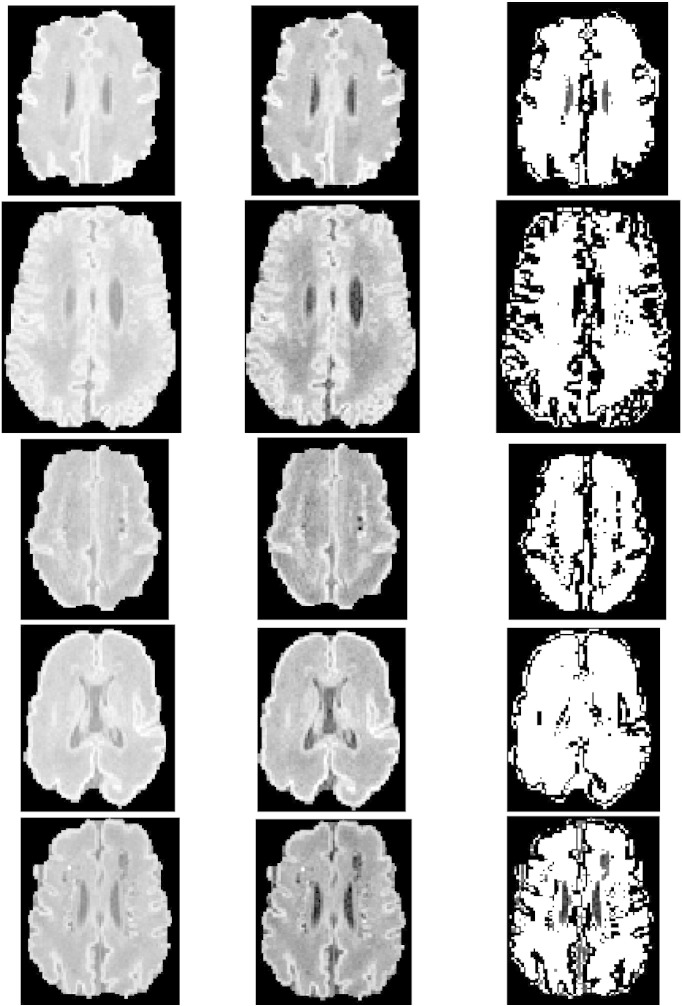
(Left column) original sections of different levels on a premature brain; (middle) enhanced MRI after pre-processing; and (right) the white matter region automatically detected.
